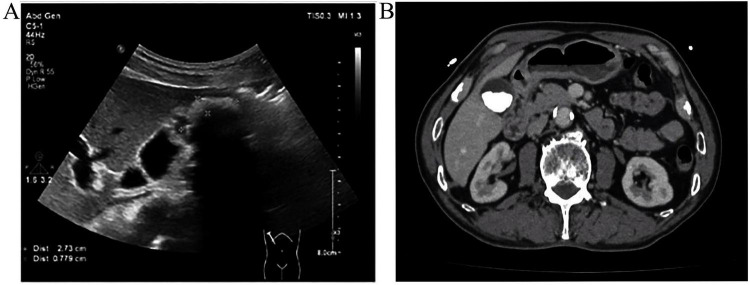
Preoperative imaging findings. (A) Ultrasonographic imaging showing hyperechoic gallstones with posterior acoustic shadowing; (B) Axial CT sections revealed the gallbladder lumen to be occupied by several fused, hyperdense foci (500–1200 HU), consistent with the subsequent macroscopic finding of ivory-white stones.
